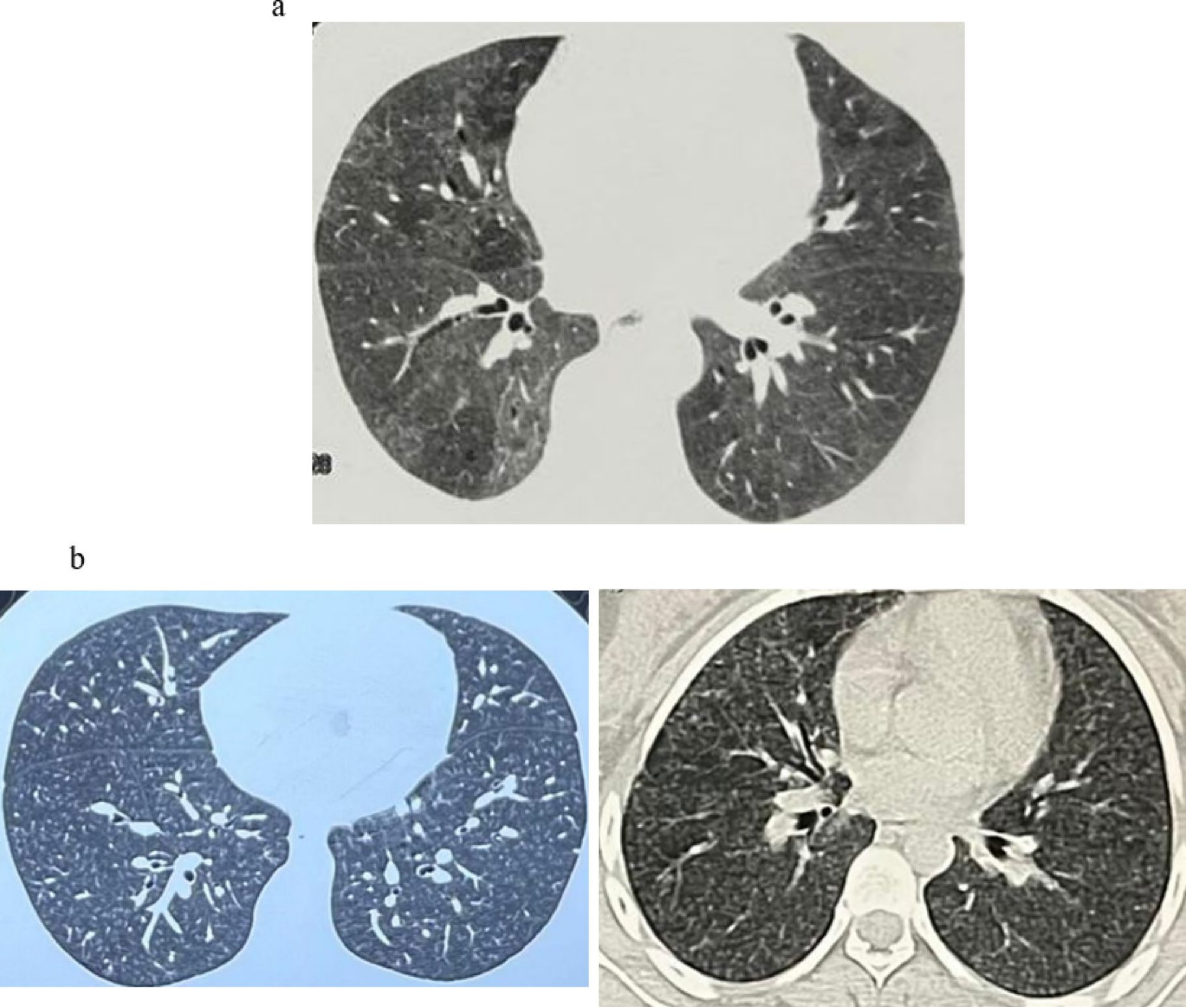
(a) HRCT scan of a patient from the study showing Mosaicism. (b) HRCT scans of patients from the study showing diffuse Centrilobular nodules.
